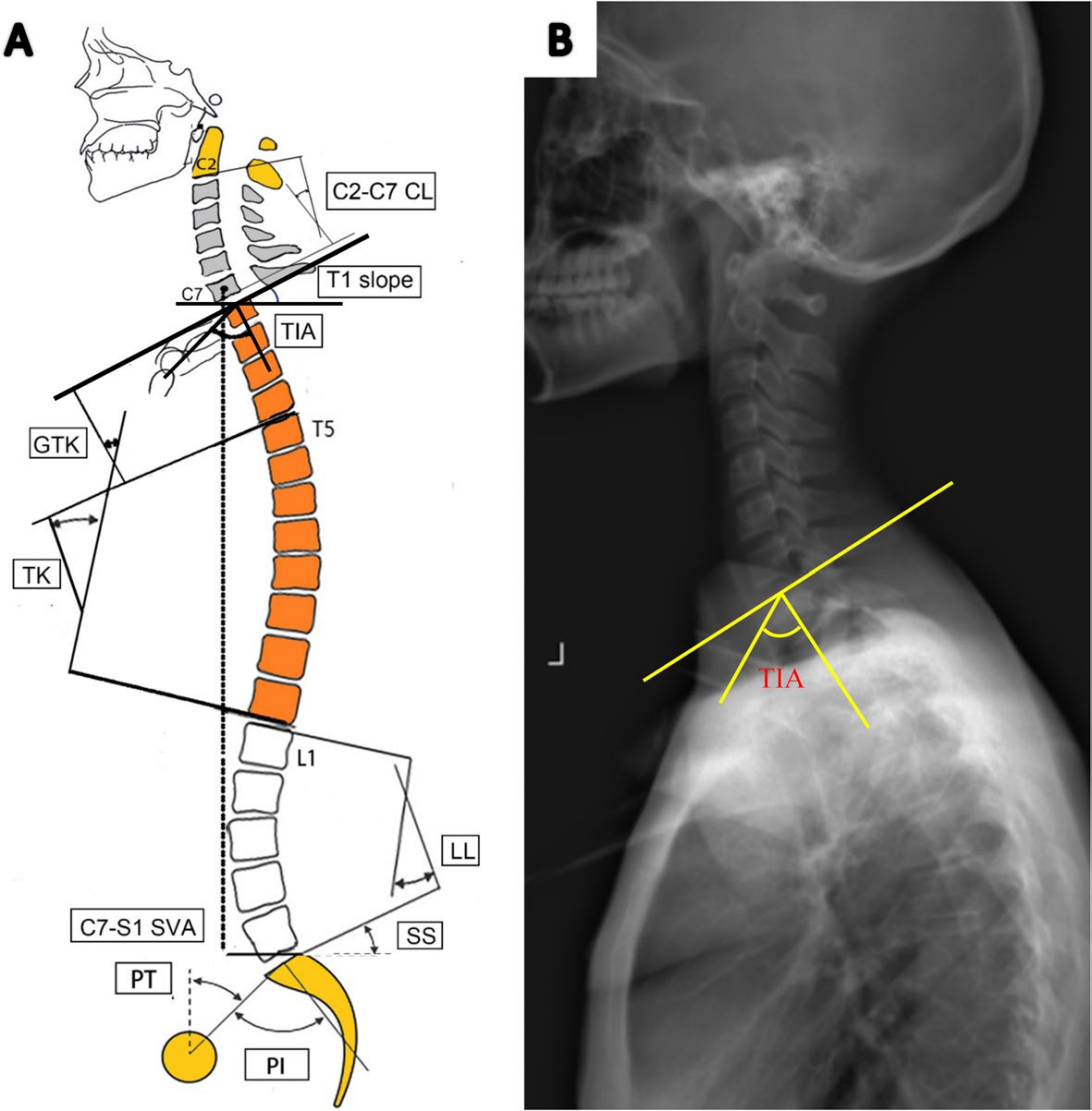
A Spinal parameters in standing lateral radiographs. T means Thoracic vertebra. TIA means Thoracic inlet angle. LL means Lumbar lordosis. TK means thoracic kyphosis. GTK means global thoracic kyphosis. PI means Pelvic incidence. PT means Pelvic tilt. SS means Sacral slope. SVA means Sagittal vertical axis. B Figure about measurement of TIA
